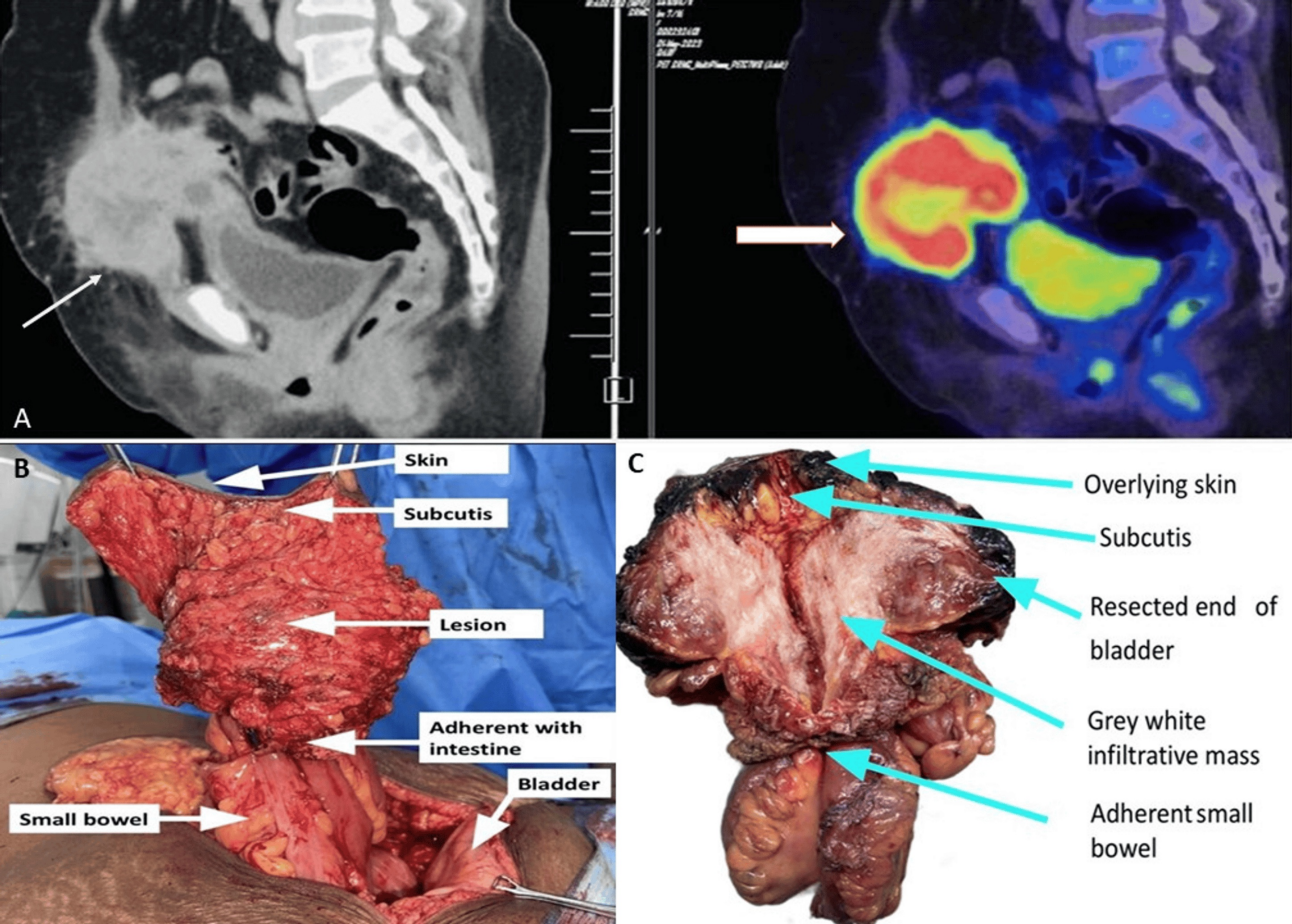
A) PET-CT image of a soft tissue mass. B) Intraoperative image of the mass. C) Serial sectioning of the mass showed a grey-white firm solid lesion. A) Both arrows pointing the heterogeneous, fluorodeoxyglucose (FDG)-avid soft tissue mass measuring approximately 70 x 76 x 90 mm (craniocaudal (CC) x anteroposterior (AP) x transverse (TR)) with irregular margins. B) Intraoperative image of the lesion adherent to the bowel wall and the abdominal wall. C) Cross-section of the mass demonstrates a grey-white firm solid irregular lesion measuring 12x8x8 cm. The mass was seen closely abutting the dome of the bladder and was seen adherent to the small bowel.
